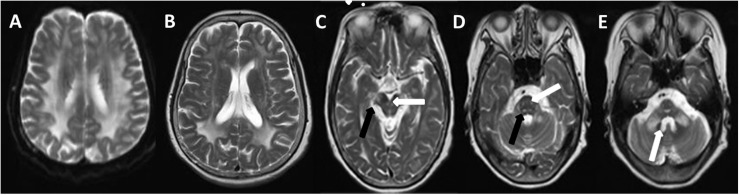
T2W (T2-weighted) changes in the proband. Diffuse hyperintensity of T2W in the white matter of frontals, parietal lobes, basal ganglia, corpus callosum, occipital lobe (A,B), mesencephalon (C, arrow), pons, and pons- and cerebellopontine-binding arms (D, arrow). The medial lemniscuses, the degustation of the superior cerebellar peduncles (C, solid arrow), pyramidal tracts, corticonuclear tracts (D, solid arrow), med peduncles, and cerebellar peduncles (E, arrow) were also affected.
